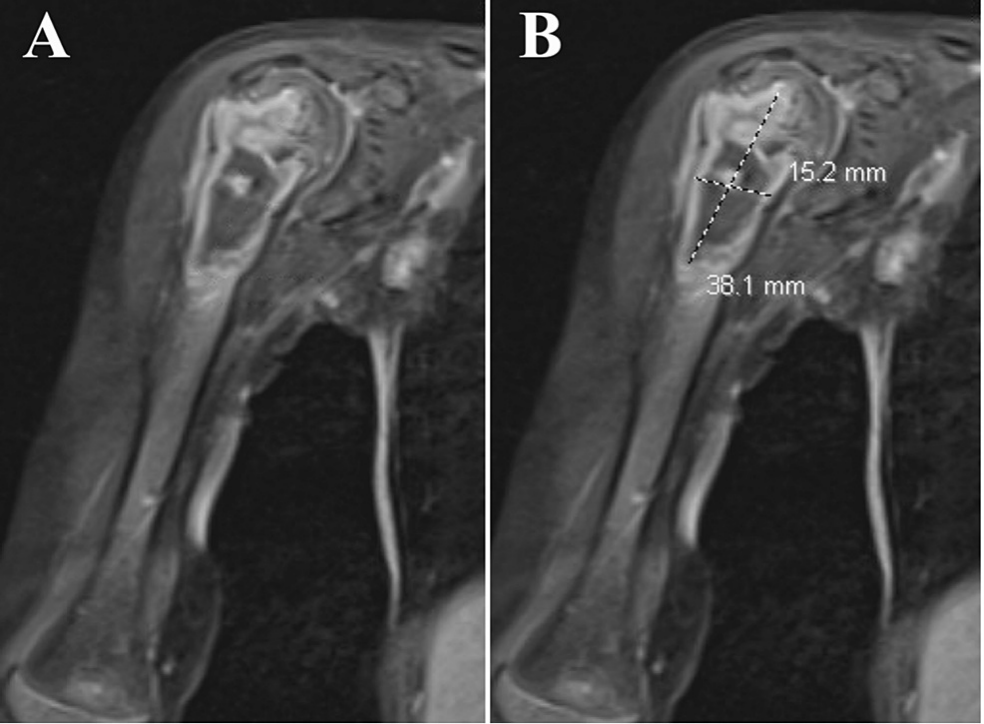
MRI of the right proximal humerus (A) with a Brodie's abscess marked (B)
